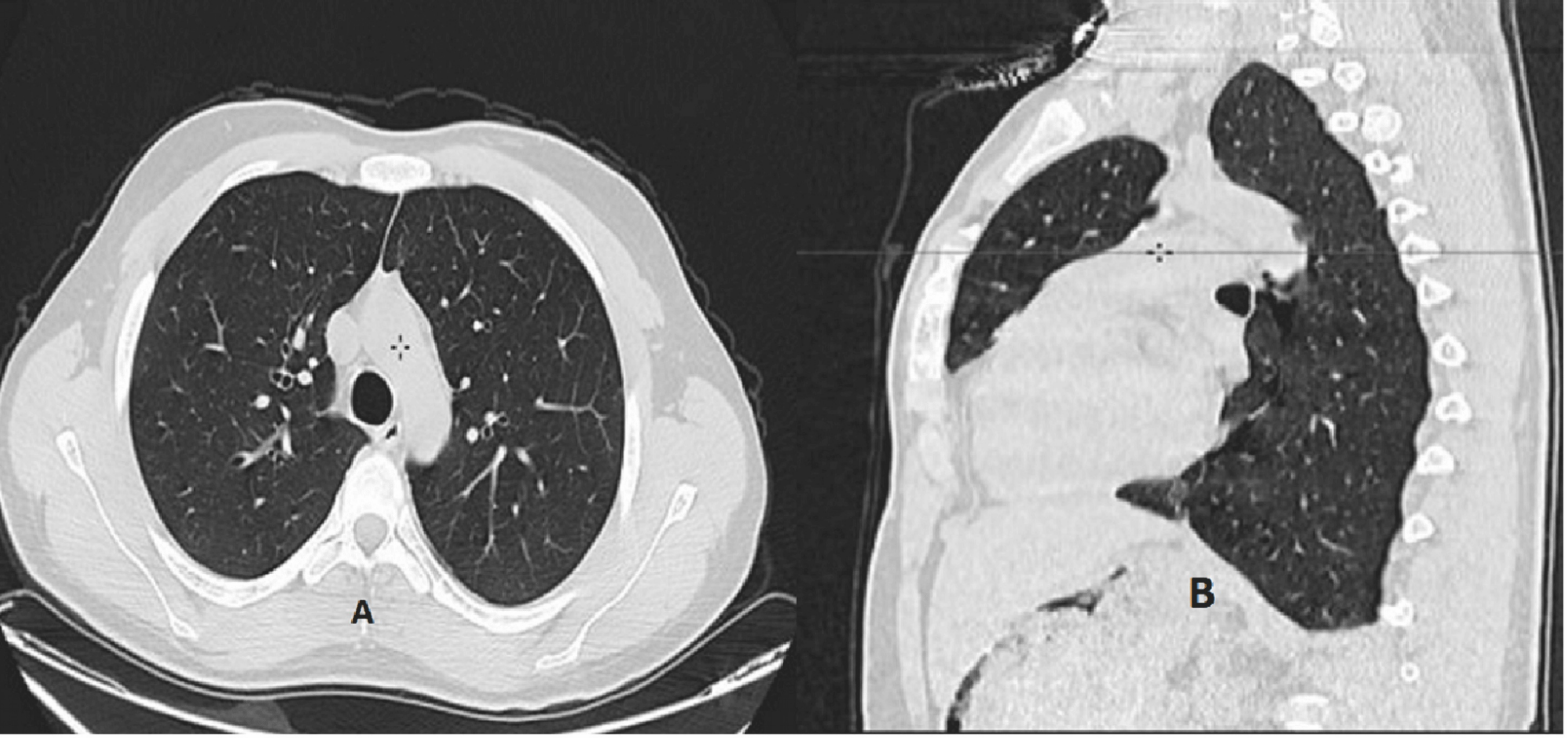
Thoracic CT imaging in axial (A) and sagittal (B) views demonstrating complete resolution of pneumothorax and pneumomediastinum.
